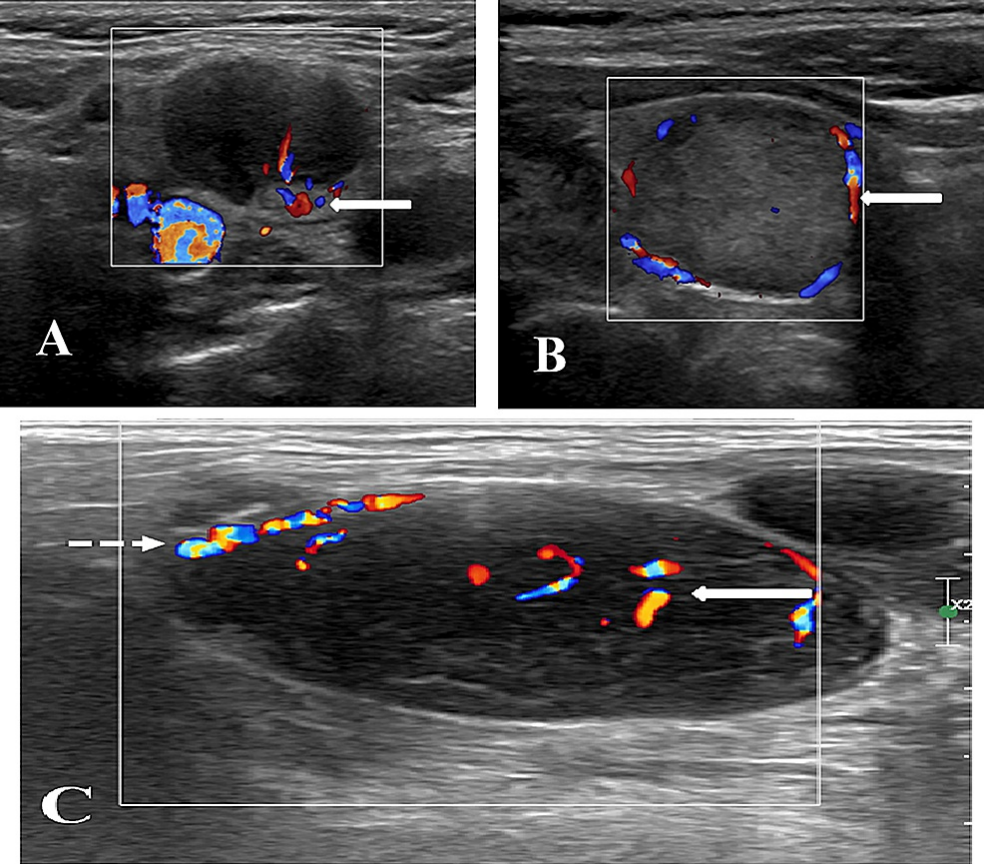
Vascular patterns on color Doppler imaging (A) Pattern 1 shows hilar vascularity (white arrow); (B) pattern 2 shows peripheral vascularity (arrow); and (C) pattern 3 shows mixed vascularity (arrow - central vascularity, dotted arrow - peripheral vascularity). Pattern 1 is a benign pattern and patterns 2 and 3 are malignant patterns.
